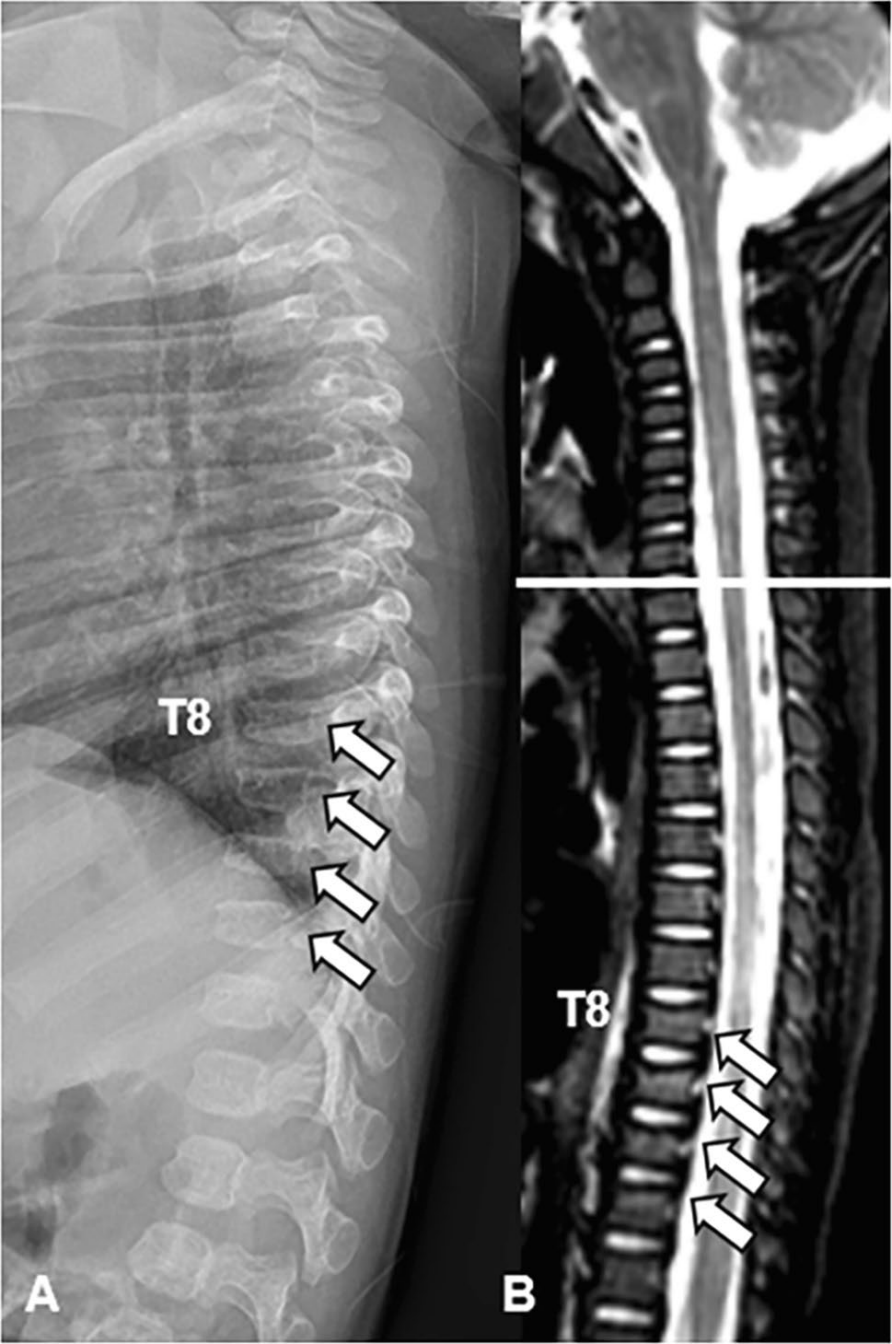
A 4-month-old male with abusive thoracolumbar compression fractures. Lateral image from a radiographic skeletal survey (A) demonstrates vertebral body height loss due to compression fractures of T8-T11 (*arrows*). Sagittal STIR MR image (B) demonstrates edema in the affected vertebral bodies (*arrows*), indicating an acute to subacute timeframe. Although there were multiple healing rib fractures (not shown), no head injuries or cervical spine injuries were present in this case (note normal cervical spine appearance above *white line* in B), highlighting the importance of whole-spine MRI
